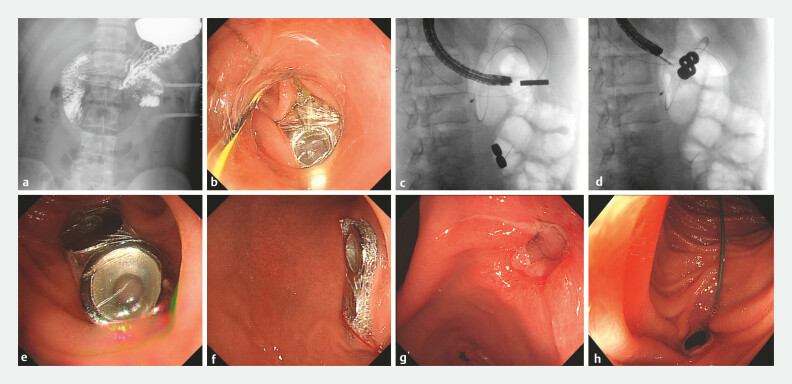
Radiographic and endoscopic images.
